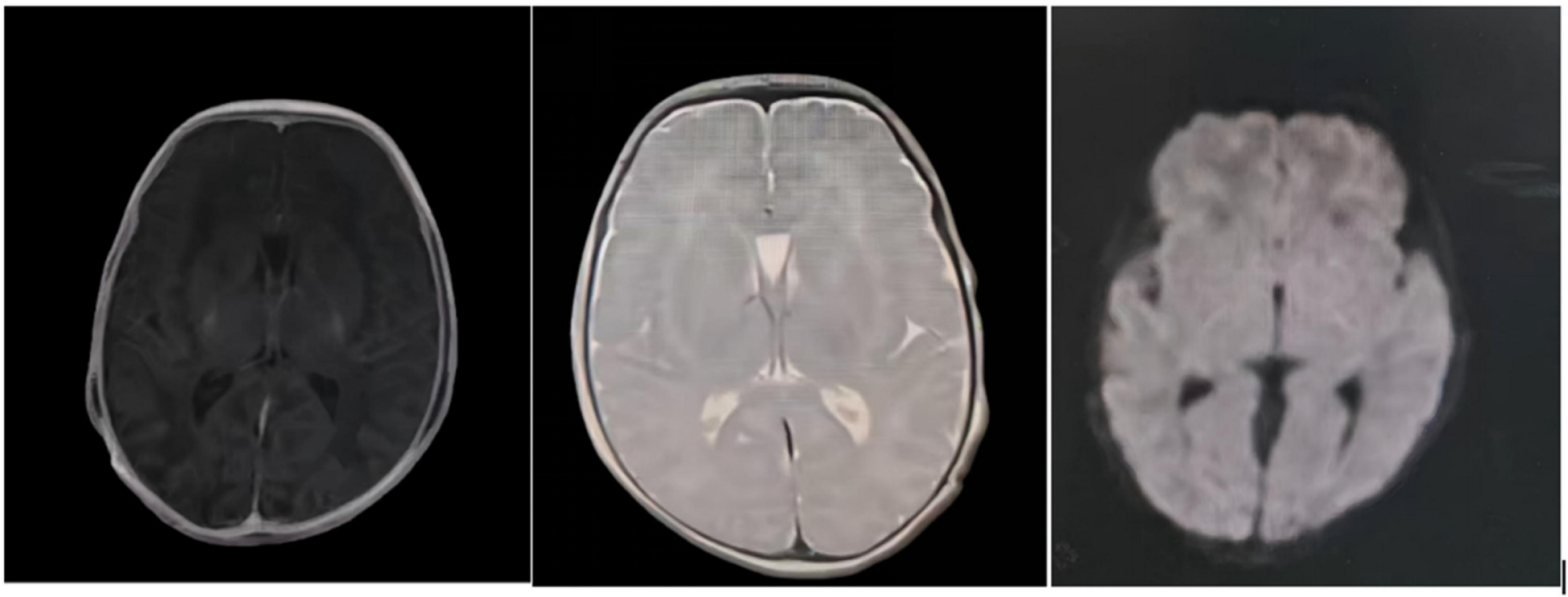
Magnetic resonance image at day 2 revealed normal.
